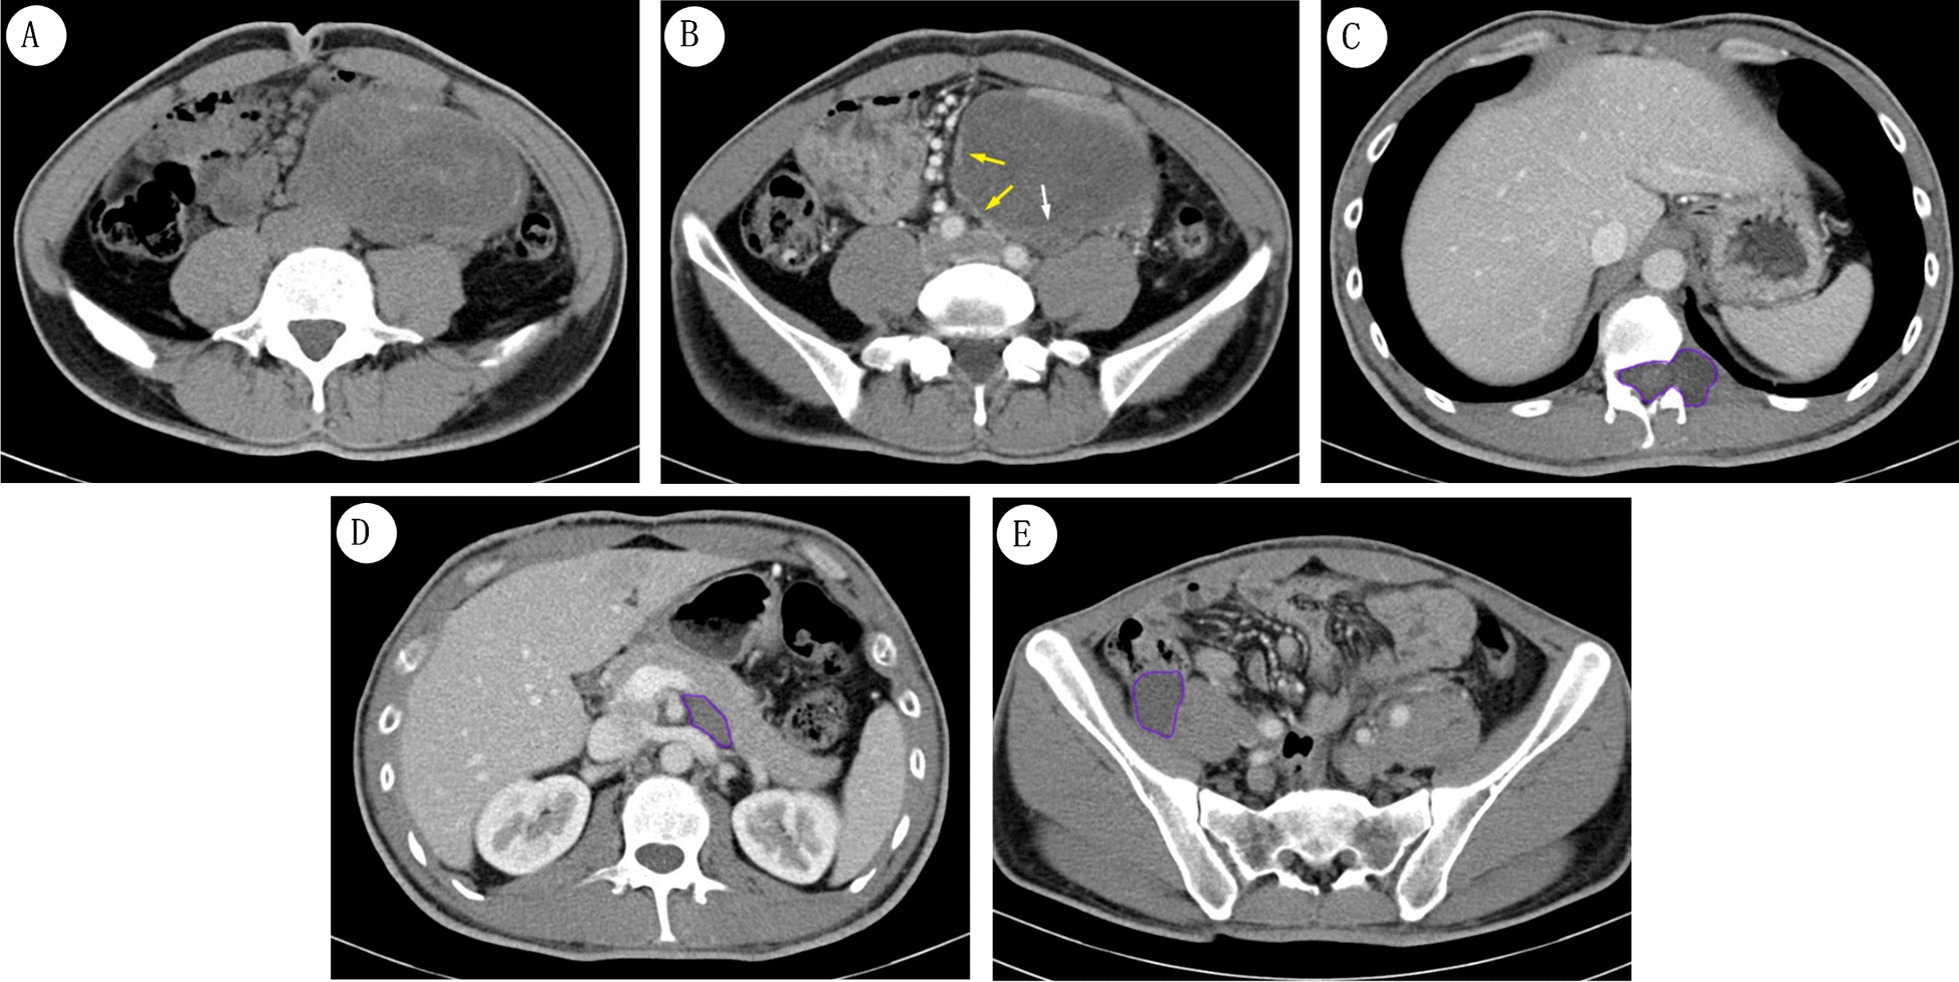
CT images of the 37-year-old male patient with a family history of NF-1. A The abdominal CT scan shows an enormous mass was in abdominal cavity. It is obvious that calcifications have occurred at the margin of mass shadow. B By contrast-enhanced CT scan, linear septum (*white arrow*) and partial edge (*yellow arrows*) are clear. In addition, A few hypodense nodules (*purple circles*) are located between the T12 and L1 (C), behind the pancreas body (D) and beside the right iliopsoas muscle (E)
